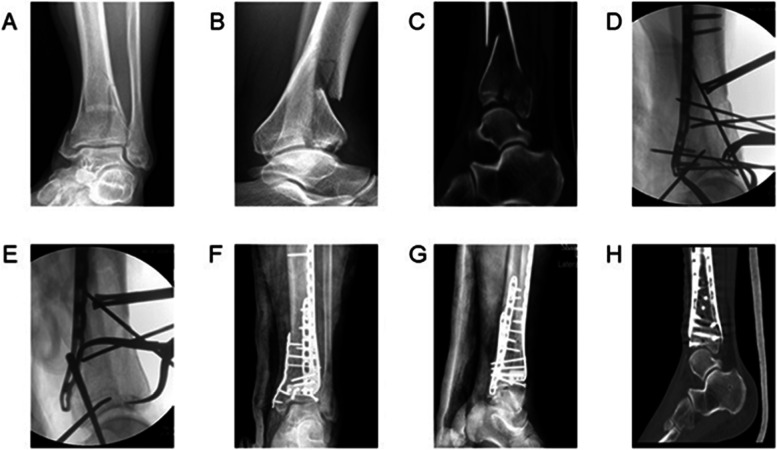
A 52-year-old man was involved in a motor vehicle accident developed AO/OTA C3 pilon fracture. A, B & C) Preoperative anteroposterior and lateral radiographs and sagittal computed tomography (CT) scans showed comminuted fracture of the posterior column articular surface. D & E) The intraoperative lateral radiographs showed adjustment of the articular surface through the combined posterolateral and anterior extensile approach, before (D) and after (E) the procedure. F & G) The postoperative anteroposterior and lateral X-rays showed good reduction and fixation. H) Postoperative sagittal CT scans showed that the reduction of the articular surface was satisfactory
